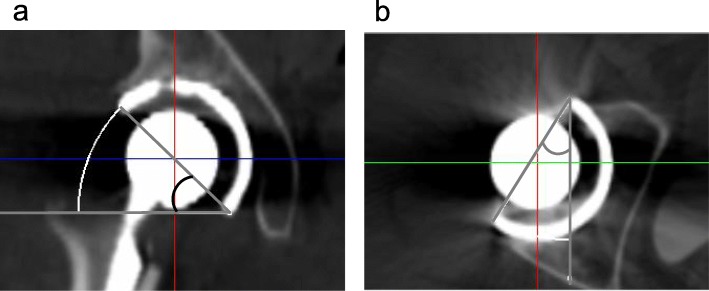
Pelvic coordinate system set to the anterior pelvic plane. Measurement of radiographic inclination on the tomographic coronal plane (a) and anatomical anteversion on the tomographic axial plane (b)
